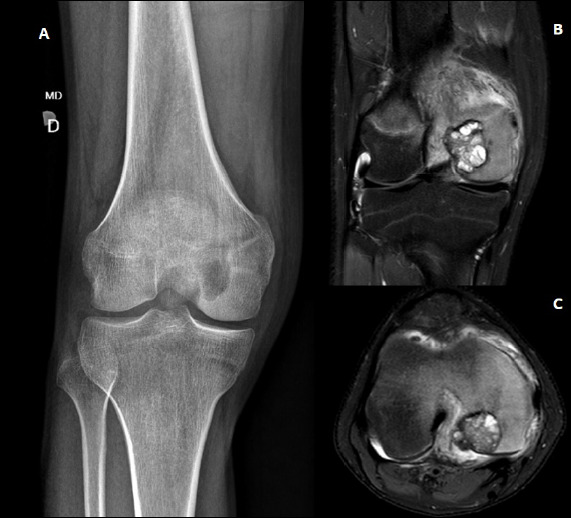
Radiography and magnetic resonance imaging of the right knee of a patient with chondroblastoma Caption A: Radiograph of the right knee of a 16-year-old boy with a history of moderate pain for six months. The bone lesion lies in the distal epiphysis of the femur, measuring 2.6 cm, totaling 16.7% of the total epiphysis size, showing an eccentric shape, in a closed physis and without a sclerosis halo or calcifications. B and C: Contrast-weighted magnetic resonance imaging with coronal and axial T2-weighted images showed heterogeneous signals, perilesional spinal cord edema, and cortical rupture in the region of the intercondyle in the axial section.
